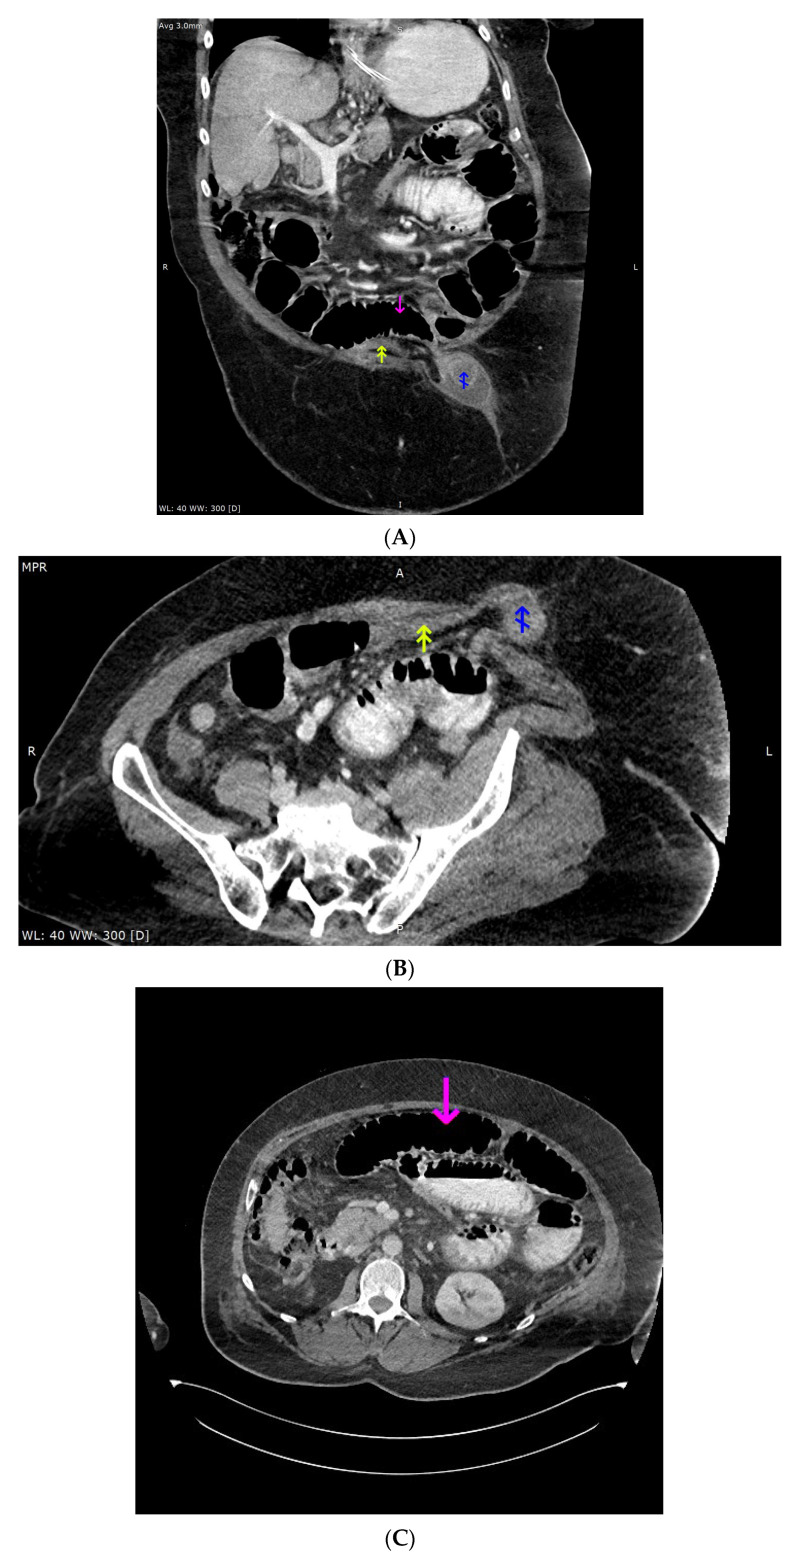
(A) Computed tomography imaging of the abdomen and pelvis, portal-venous phase, oral contrast administered, oblique (closest to coronal) reconstruction (3 mm). Magenta arrow points to the pre-stenotic, dilated small intestine, lumen mostly filled with gas; double yellow arrow points to the post-stenotic, small intestine without any content; blue arrow points to the edematous small intestine loop inside the hernia sac, surrounded by fluid; (B) Computed tomography imaging of the abdomen and pelvis, portal-venous phase, oral contrast administered, oblique (closest to axial) reconstruction (3 mm). Double yellow arrow points to the post-stenotic, small intestine without any content; blue arrow points to the edematous small intestine loop inside the hernia sac, surrounded by fluid. (C) Computed tomography imaging of the abdomen and pelvis, portal-venous phase, oral contrast administered, axial reconstruction (1.5 mm). Magenta arrow points to the pre-stenotic, dilated small intestine, lumen mostly filled with gas.
